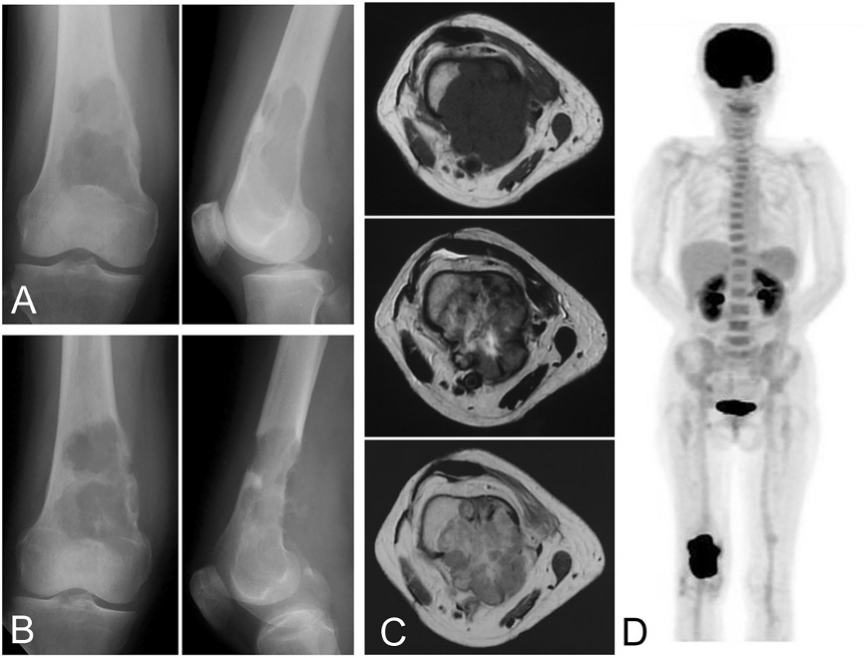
Epithelioid angiosarcoma images. (A) Plain X-radiographs show an osteolytic lesion in the distal femur. (B) This lesion has apparently widened after 1 month. (C) Magnetic resonance imaging shows a lobulated lesion with a low signal on T1-weighted imaging (top) and a heterogeneous signal on T2-weighted imaging (middle), and heterogeneous gadolinium enhancement is observed on T1-weighted imaging (bottom). (D) [^18^F]-2-fluoro-2-deoxy-D-glucose positron emission tomography reveals a solitary lesion in the distal femur.
